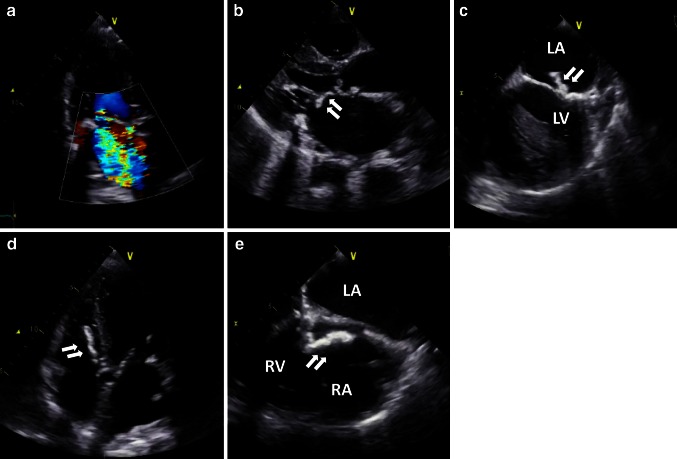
Transthoracic echocardiography (TTE): color doppler image showing severe mitral regurgitation (a), parasternal long-axis (b) and apical four-chamber view (d). Transesophageal echocardiography (TEE): parasternal long-axis (c) and apical four-chamber view (e). *RA* right atrium; *RV* right ventricle; *LA* left atrium; *LV* left ventricle. *White arrows* indicate a mobile, highly echoic mass
